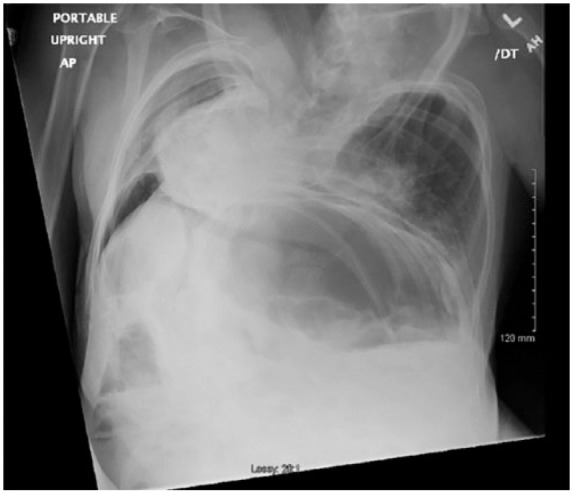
Chest X-ray showing left basilar airspace disease.
